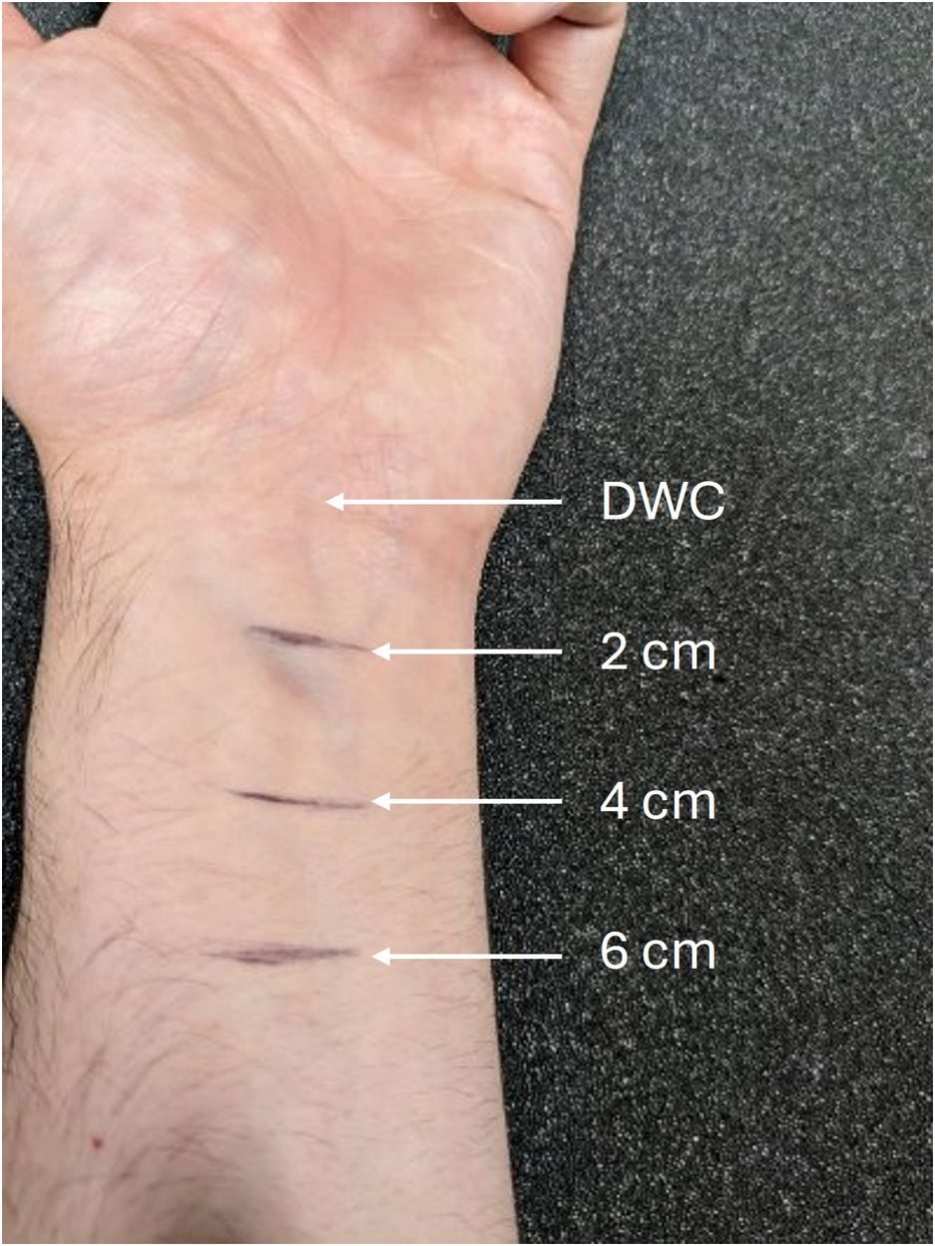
Participant’s left arm. The supinated arm rested on a flat surface. Three lines were drawn at 2 *cm*, 4 *cm* and 6 *cm* from the DWC. Cross-sectional ultrasound imaging was performed at these three lines.
